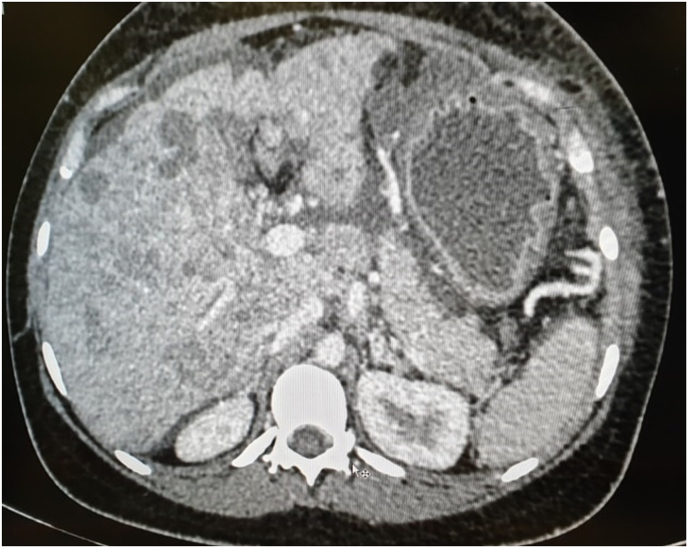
CT Venous phase. Legend: Ascites.
